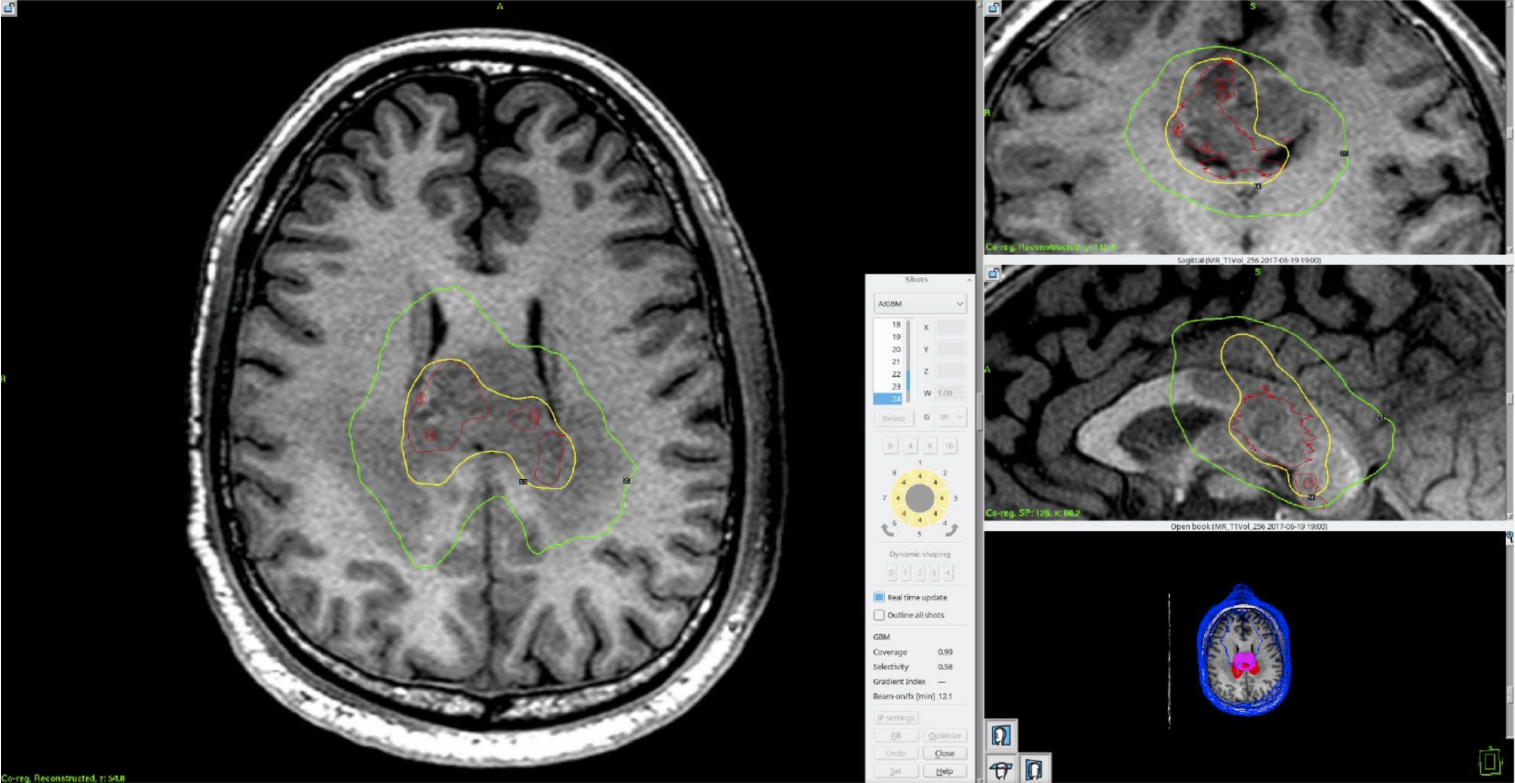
Screenshot obtained from GammaPlan version 11.1.1 treatment planning software containing volumetric T1-post contrast MRI brain images after upfront surgical debulking for a selected patient receiving fractionated radiosurgery with GammaKnife ICON. Outlined in red is the post-operative intended treatment volume as determined by fusion with pre-operative imaging as well as close collaboration with the neurosurgical team to determine residual disease versus post-operative changes (15.5 cc volume). Yellow and green represent the 50% and 20% isodose lines, respectively. Dose was prescribed to the 50% isodose line (yellow). As outlined, coverage of the treatment volume was 99% with a 58% selectivity and beam-on time of 12.1 min per fraction
